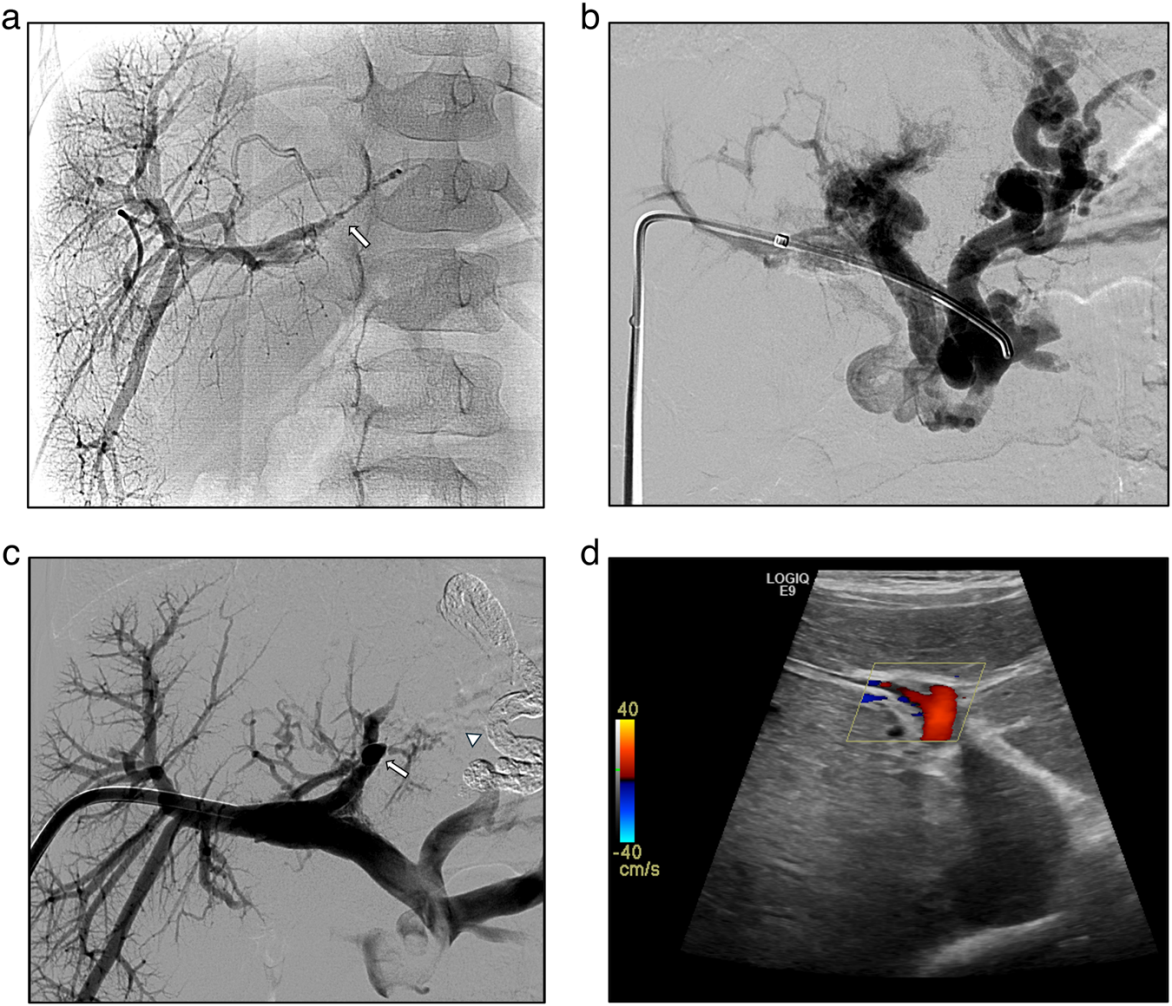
A 4-year-old male with a history of perinatal umbilical vein catheterization presented with severe hematemesis. a shows percutaneous transhepatic portal venography with opacification of right intrahepatic portal branches and partial thrombosis of the Rex recessus (arrow). A transhepatic retrograde recanalization of the extrahepatic portal tract was performed. b shows spleno-mesenteric venography with cavernous transformation and left gastric varices. c shows the successful complete recanalization of the portal system, including the Rex recessus, and variceal glue embolization (arrowhead). Gastric varices disappeared at the endoscopic examination performed after 3 months. Platelet count normalization and portal vein patency at colour-Doppler ultrasound (d) were maintained during the 6-month follow-up
